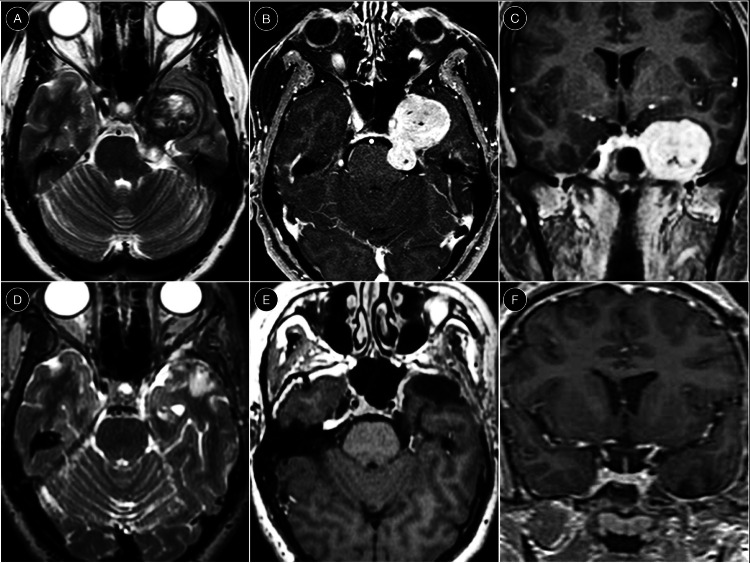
A-C Preoperative axial T2-weighted MRI (A), and axial (B) and coronal (C) T1-weighted gadolinium-enhanced MRI. D-F Postoperative axial T2-weighted (D) and axial (E) and coronal (F) T1-weighted gadolinium-enhanced MRI demonstrating the extent of resection
